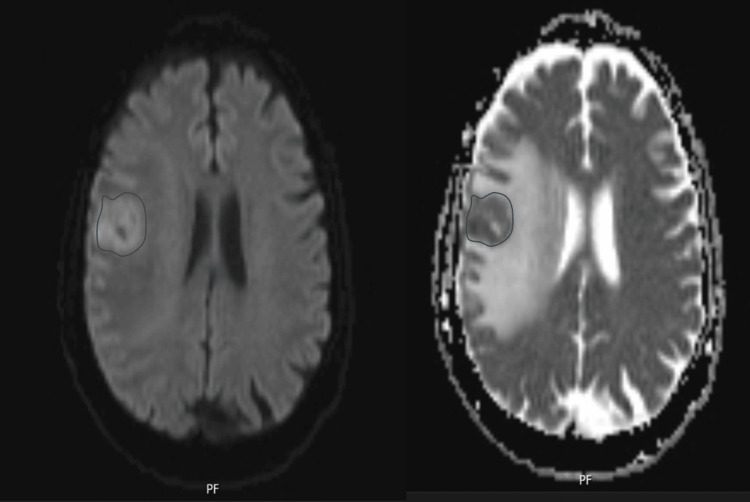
Diffusion-weighted imaging (DWI) MRI demonstrating restricted diffusion. Diffusion-weighted imaging (DWI) Mmagnetic resonance imaging (MRI) demonstrating restricted diffusion in a right frontal cortico-subcortical lesion measuring 34 × 36 × 34 mm. The area of diffusion restriction is indicated by a blue circle. The mean ADC value is approximately 0.9 × 10⁻³ mm²/s, indicating low diffusion.
